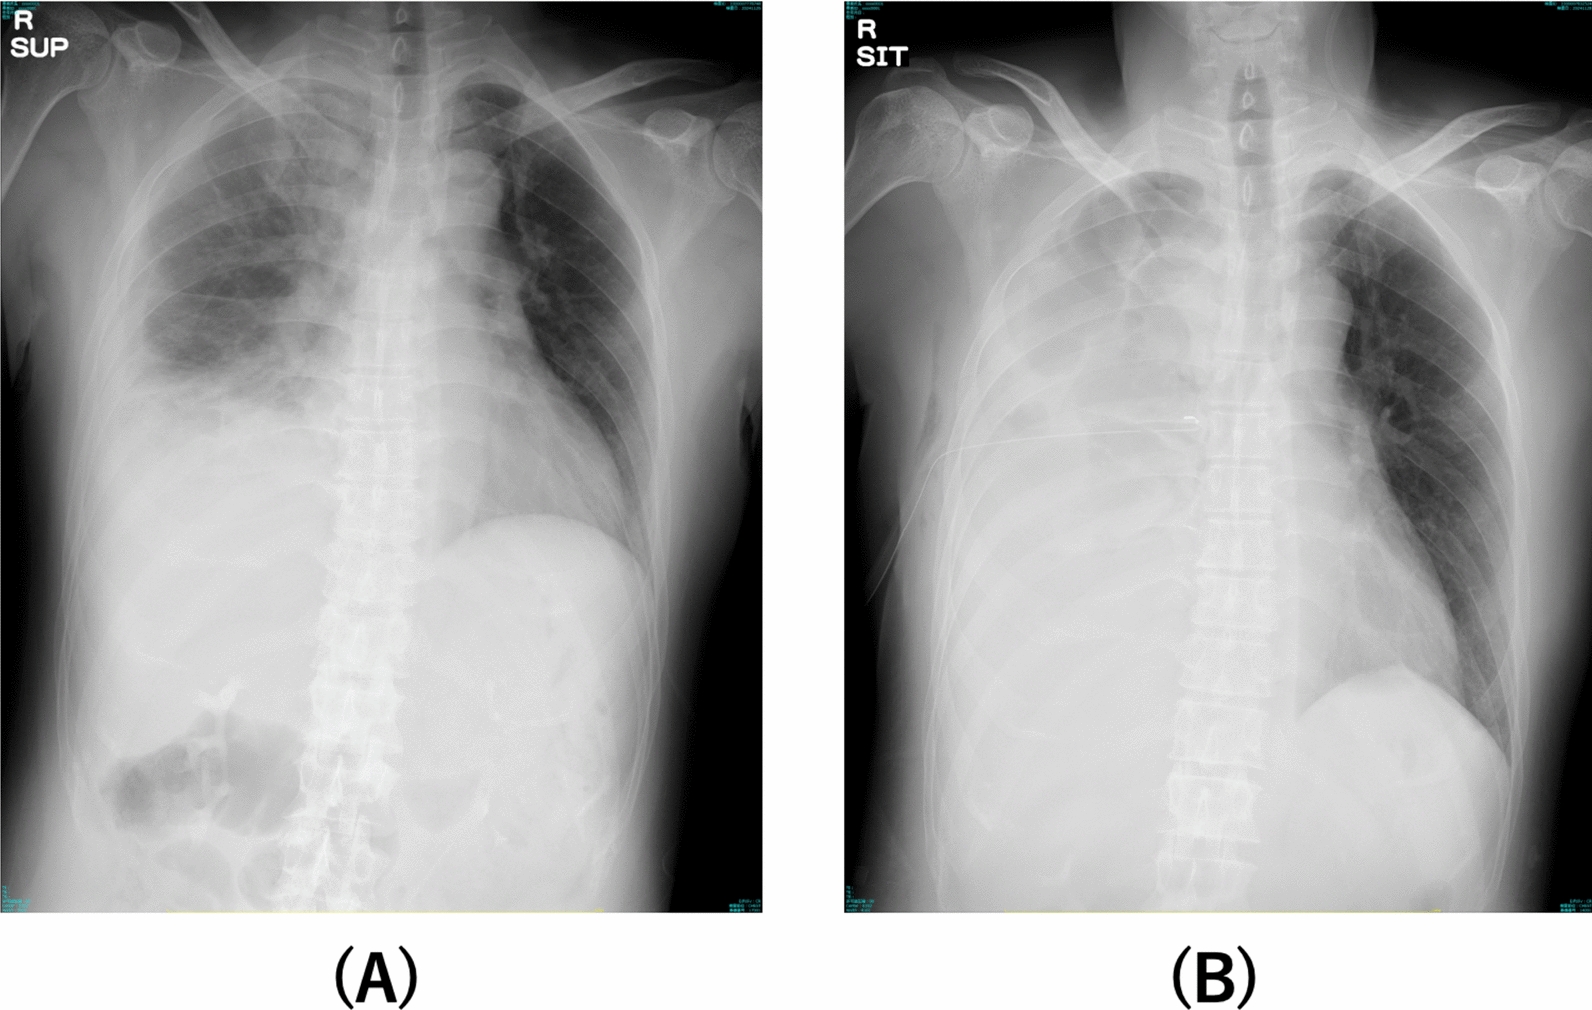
Comparison of chest radiography findings on admission (a) and day 3 (b) shows significant increase in right pleural effusion
